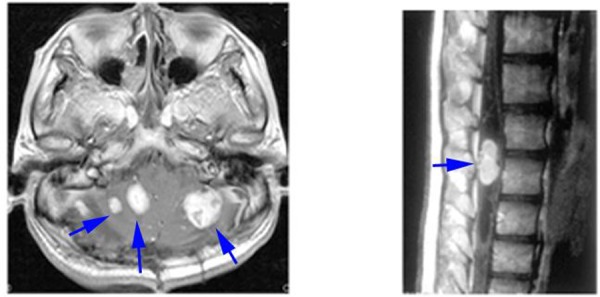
Contrast-enhanced T1 weighted MRI of CNS HBs in VHL. Left, multi-cerebellar HBs; right, lumbar spinal cord HB with syrinx.
